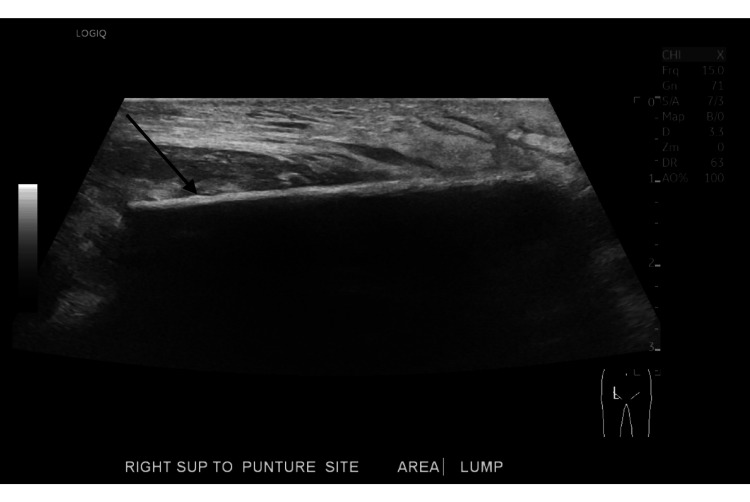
Ultrasound demonstrating retained wooden foreign body (day 10) Longitudinal ultrasound image of the right thigh obtained on the third presentation, showing a linear echogenic structure (arrow) within the deep subcutaneous tissues with posterior acoustic shadowing and an adjacent fluid collection consistent with abscess. The image was acquired using a high‑frequency linear transducer (e.g., 10-15 MHz) at a depth of approximately 3-4 cm.
